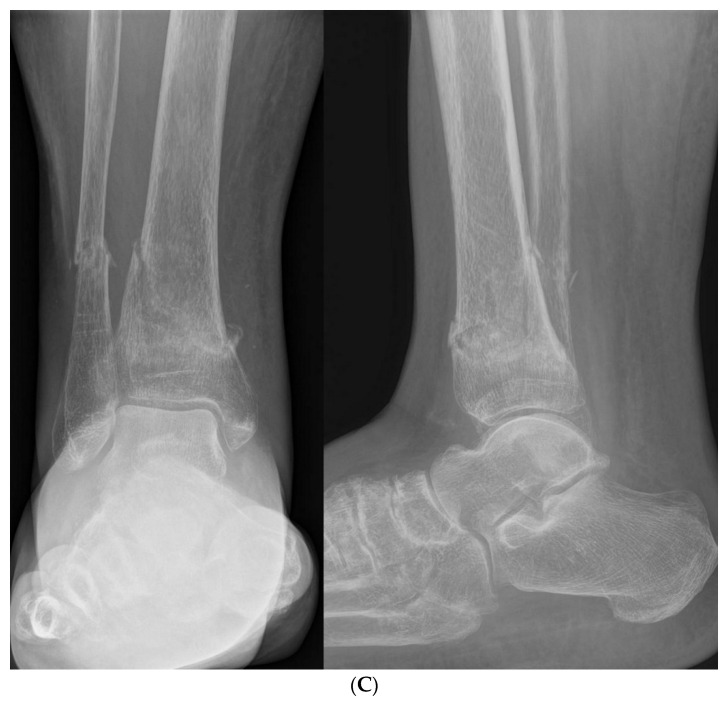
(A) Examples of pathological findings in adults (in comparison with the normal conditions in children from Figure 5). (a) Suprapatellar recess of a 48-year-old male with moderately severe inflammation due to gout. Visible folds of thickened synovium and a moderately increased amount of fluid. Linear probe 6–12 MHz. (b) Power Doppler image showing an uneven cortical layer of the femur in a 75-year-old male with multiple metastases of prostate cancer in the bones (the patient did not know about his disease until the day of ultrasound examination of the limbs). Visible increased vascularization of the periosteum. Linear probe 3–12 MHz. (c) Damage to the subscapularis tendon in a 58-year-old male; the distance to which the tendon has contracted is marked with a dotted line, the hypoechoic areas in the lesion zone correspond to traces of fluid and folds of the thickened synovial membrane inverting into the area of the lesion. 3–12 MHz linear probe. (d) Stress fracture of the tibia in a 61-year-old woman: visible discontinuity of the cortical layer, thickening of the periosteum, and callus formation. Linear probe 3–12 MHz. (B) X-ray images of the right (on the left) and left (on the right) femurs of a patient whose US images are presented in Figure 6(Ab). Visible osteolytic and osteosclerotic metastatic lesions in both femurs. (C) Anteroposterior and lateral X-ray images of the patient described in Figure 6(Ad). Patient presented with exacerbating pain in the distal 1/3 of the lower leg. There was no history of trauma.
